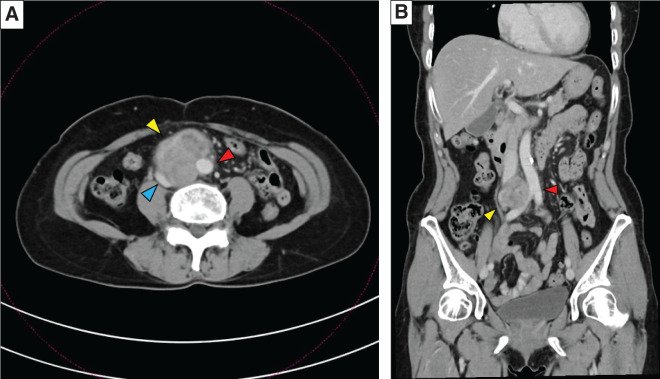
(A), (B). Abdominal computed tomogram images showed a 7.0 × 5.3 cm multilobulated, heterogeneous, soft tissue mass (yellow arrowhead) in the right hemiabdomen, encasing the inferior vena cava (blue arrowhead) and partially encasing the descending abdominal aorta (red arrowhead).
